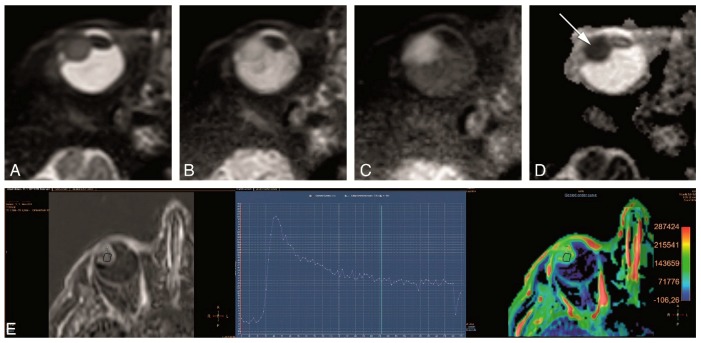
(A–E). Study protocol: Functional axial MRI sequences in the same patient as in Figure 2. (A–D) TSE DWI, with b values of 0 s/mm^2^ (A), 400 s/mm^2^ (B), 800 s/mm^2^ (C) and Apparent Diffusion Coefficient (ADC) (D). Restricted diffusion in the uveal melanoma (arrow). (E) Dynamic Contrast-Enhanced (DCE) with a good quality, no movement artefacts, showing a wash-out TIC pattern.
